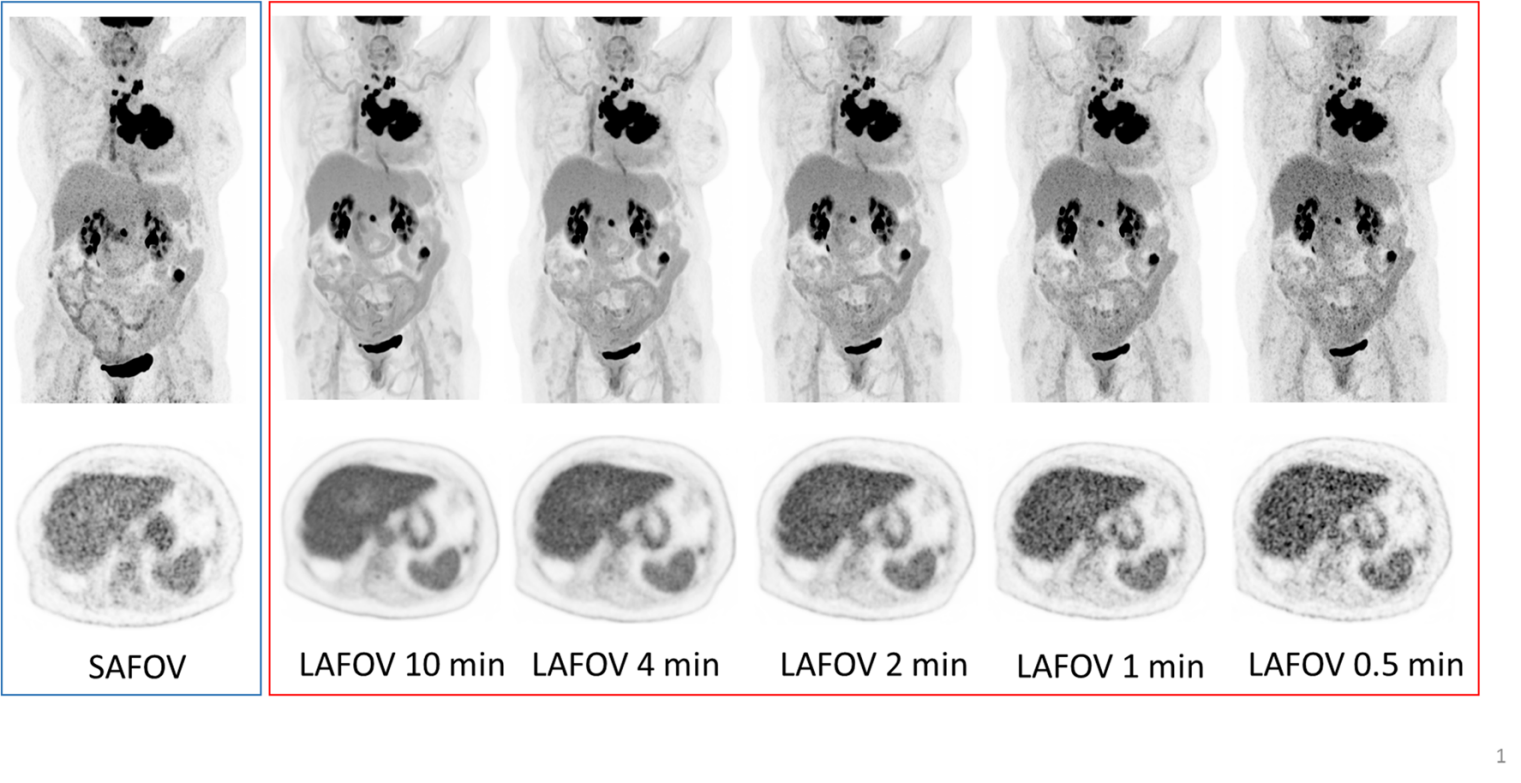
Example maximum intensity projection (MIP, top row) and axial PET images (bottom row) images for a 57-year-old female with non-small cell lung cancer, presented are images for the regular SAFOV acquisitions on the left (blue margin) and the LAFOV for 10-, 4-, 2-, 1- and 0.5-min acquisitions (right, red margin). For reference, the PET window is set to 0 to 8.5 SUV
